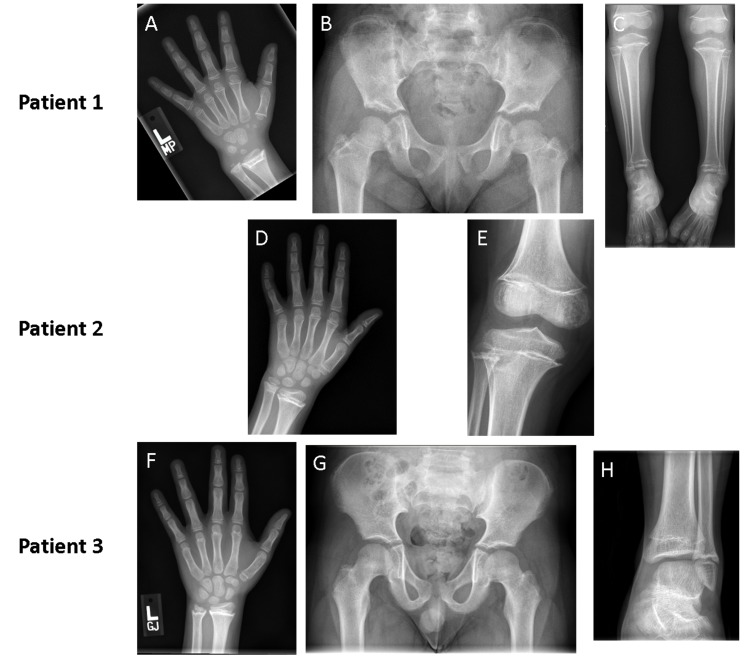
Imaging of patients at follow up showing skeletal deformities resulting from widespread physeal fusions Radiographs of patient 1 at 18 months after cessation of therapy show protrusion of the ulnar head and widespread physeal fusion in hand bones (Panel A); abnormally short femoral necks (Panel B); and varus ankles, with widespread physeal fusions of bones in the lower leg (Panel C). Radiographs of patient 2 at 14 months after completion of vismodegib therapy show widespread physeal fusions in hand and forearm bones (Panel D) accompanied by sclerotic thickening in all physes of the knee (Panel E). Three radiographs of patient 3 taken 16 months after cessation of therapy show abnormalities that are less prominent but similar to those of patient 1. Advanced bone age (Panel F), shortened femoral necks (Panel G), and distal fusion of the tibia with relative sparing of the fibula (Panel H) was seen.
